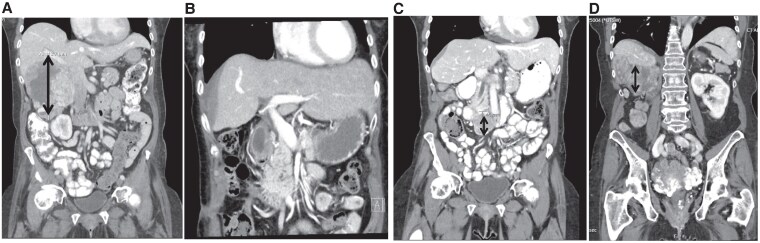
Computed tomography of the abdomen and pelvis showing the adrenal mass across the clinical course. (A) At the initial diagnosis; (B) After right adrenalectomy, nephrectomy, and partial hepatectomy; (C) At the detection of recurrent aortocaval node; (D) At the onset of non-islet cell tumor hypoglycemia. The double-sided arrow indicates the location of the adrenal mass at each time point.
